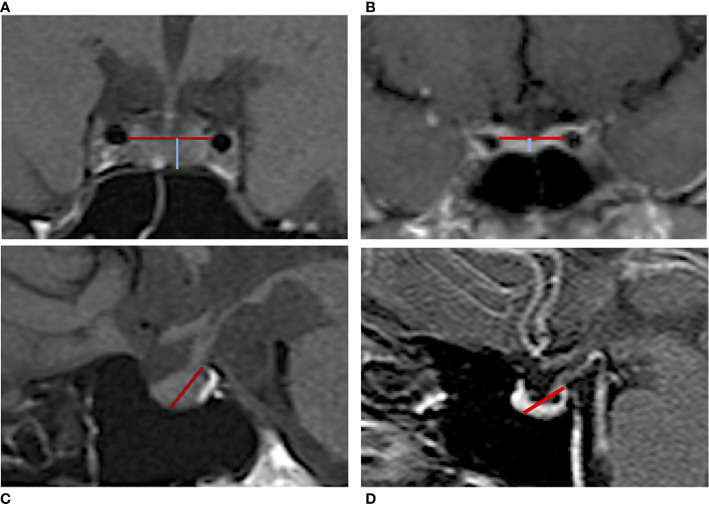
Manual measurement of the pituitary gland in MRI T1-weighted images. Coronal scan of an IIH patient (A) without ES and (B) with ES. The blue line reflects the gland height (cranio-caudal diameter) defined as the distance between the inferior edge of the *sella turcica* and the superior edge of the pituitary gland; the red line indicates the pituitary gland thickness (latero-lateral diameter) that was measured as the maximum transverse diameter. Sagittal scan of an IIH patient (C) without ES and (D) with ES. The red line indicates the pituitary length (anterior-posterior diameter) measured from the posterior clinoid process to the middle point of the pituitary fossa.
